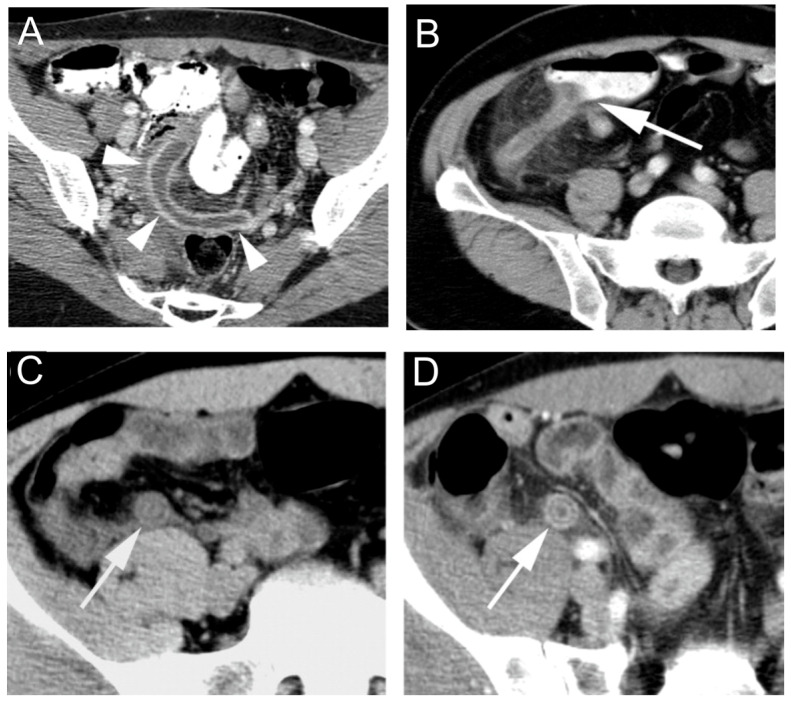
Computed tomography findings of appendicitis. (A) Axial CT image showing an enlarged, fluid-filled appendix (arrowheads). (B) Axial CT image showing edema of the cecal tip with oral contrast pointing (arrow) towards the base of the inflamed appendix. Axial CT images in the same patient (C) before and (D) after intravenous contrast show a thickened appendix with submucosal edema. Reprinted with permission of [138].
